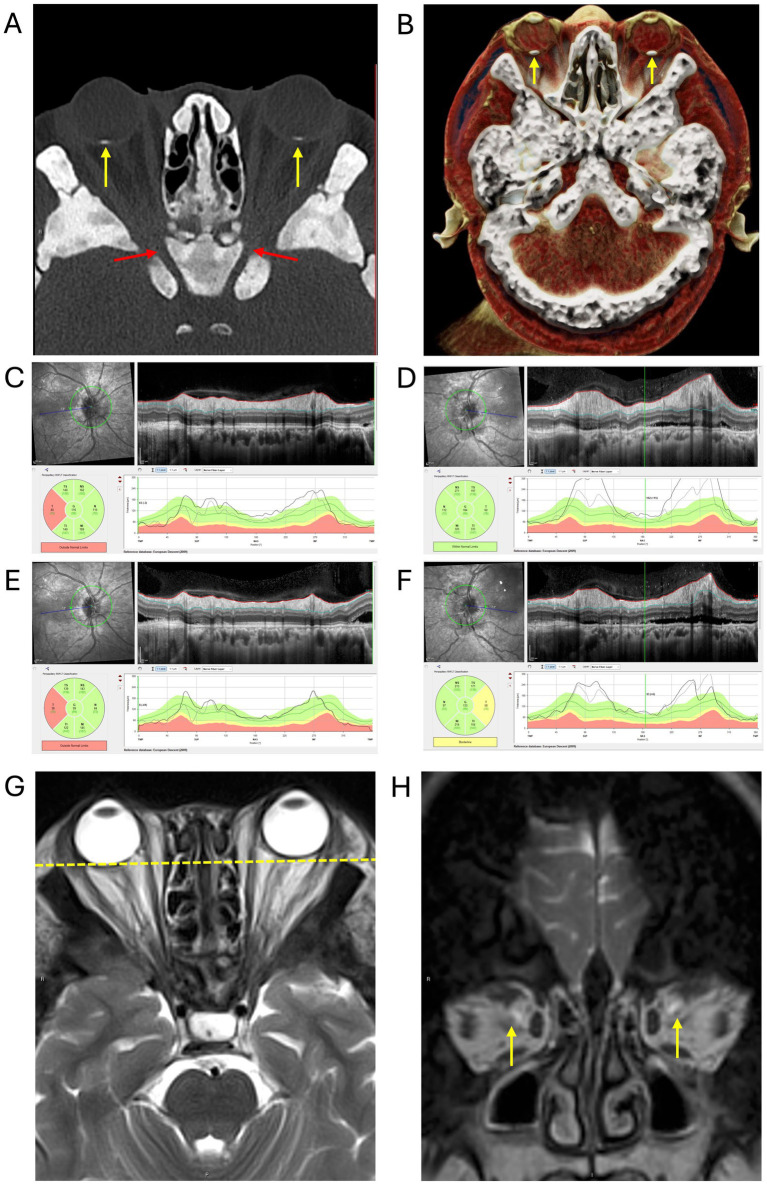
(A) CT head showing bilateral optic canal narrowing (red arrows) and bilateral choroidal osteomas (yellow arrows). (B) CT volume rendering technique showing extensive cranial hyperostosis and bilateral choroidal osteomas (yellow arrows). (C) OCT retinal nerve fibre layer scan showing increase in retinal nerve fibre layer thickness right eye. (D) OCT retinal nerve fibre layer scan showing increase in retinal nerve fibre layer thickness left eye and small amount of peripapillary subretinal fluid. (E) Reduction in right eye retinal nerve fibre layer thickness following acetazolamide 250 mg twice daily. (F) Reduction in left eye retinal nerve fibre layer thickness following acetazolamide 250 mg twice daily. Of note there is peripapillary subretinal fluid present (most likely due to macular subretinal fluid), which would impact the total retinal thickness measurement. However, the retinal nerve fibre layer thickness used as measure for papilloedema in this case was not influenced by peripapillary fluid as evidenced by satisfactory RNFL segmentation. RNFL segmentation was checked for all RNFL scans and if segmentation error present, manual resegmentation was performed. (G) Axial MRI T2 weighted imaging showing bilateral proptosis (yellow dashed line). (H) Coronal MRI T2 weighted imaging showing bilateral optic nerve atrophy (yellow arrows).
